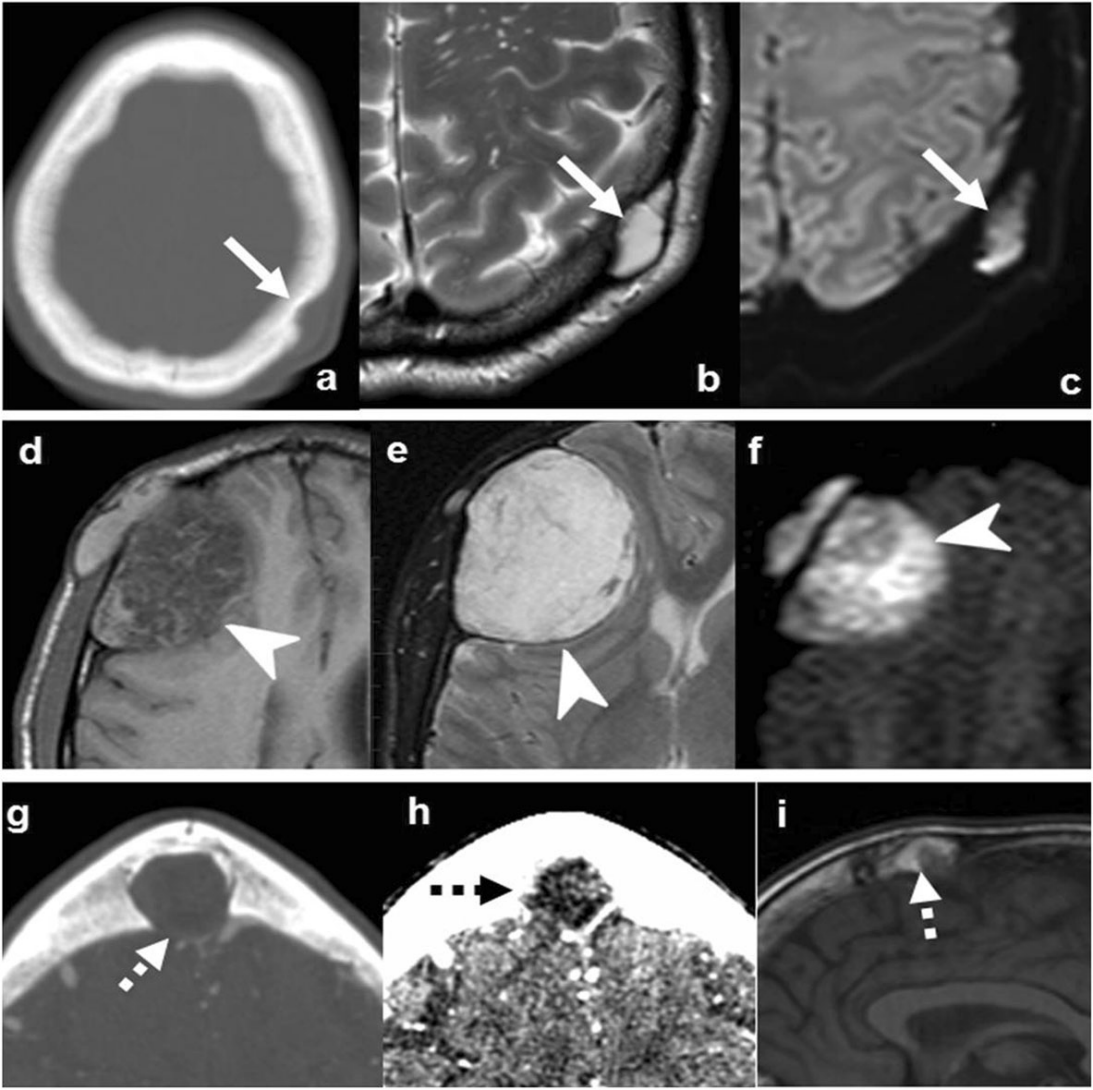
Epidermoid cysts (a–c and d–f) and dermoid cyst (g–i). Case 1 (a–c): Extracranial soft-tissue mass (arrows) remodeling the outer table on NECT (a), with fluid T2-signal (b), and markedly restricted diffusion (*b* = 1000) (c). Case 2 (d–f): Transdiploic mass (arrowheads), predominantly intracranial, T1WI (d) and T2WI (e) heterogenous and intense diffusion restriction (f). Case 3 (g–i). Frontal skull lesion (dashed arrows) disrupting the inner table on CT (g, h); content is CT-hypodense and T1-hyperintense, corresponding to fat (i)
